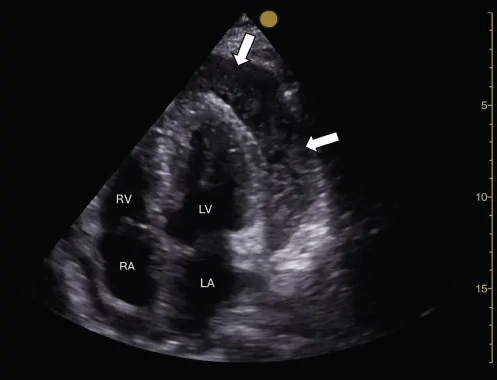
Point-of-care ultrasound showing an apical four chamber view of the patient’s heart with visualization of the left atrium (LA), left ventricle (LV), right atrium (RA), and right ventricle (RV). Note the large mixed-echogenicity pericardial effusion (white arrows).
